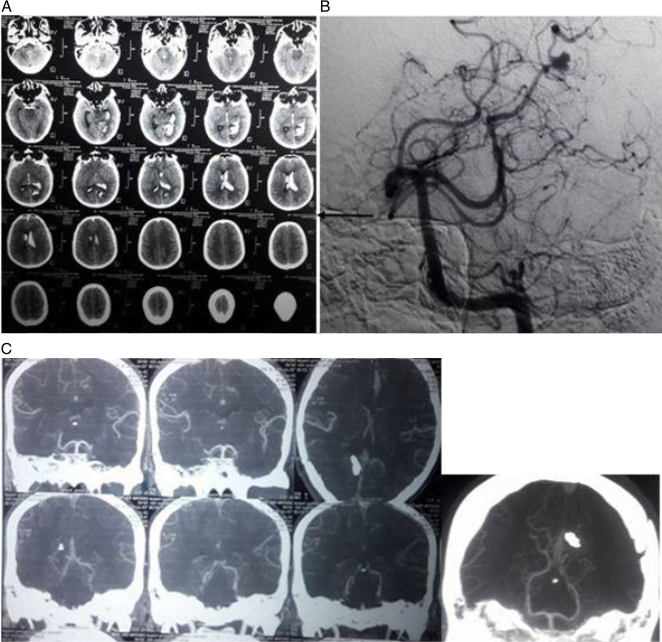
Plain CT head (A) of a 25-year-old male who presented with acute onset severe headache of 24 h duration. Digital subtraction angiography (B) shows a bi-lobed aneurysm in the P4 segment of the left posterior cerebral artery (arrow). This patient underwent trapping of the aneurysm as clip placement without significantly narrowing the parent vessel was impossible during surgery. Postoperative CT angiography (C) shows complete obliteration of the aneurysm. The patient had no neurological complications including intact visual fields postoperatively. CT, computed tomography.
